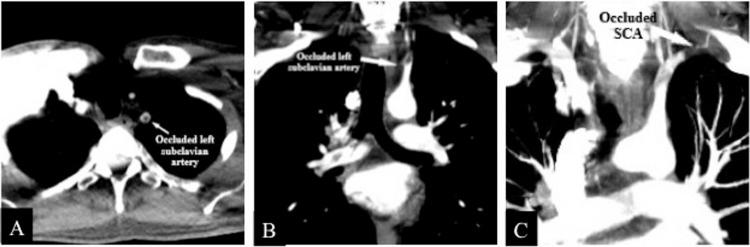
CTA findings of the left SCA occlusion. A: Filling defect in the left SCA, B: Occlusion of the left SCA, C: Partial thrombus before the left vertebral artery in the left SCA, total occlusion after the vertebral artery, patent the left vertebral artery. SCA: subclavian artery
